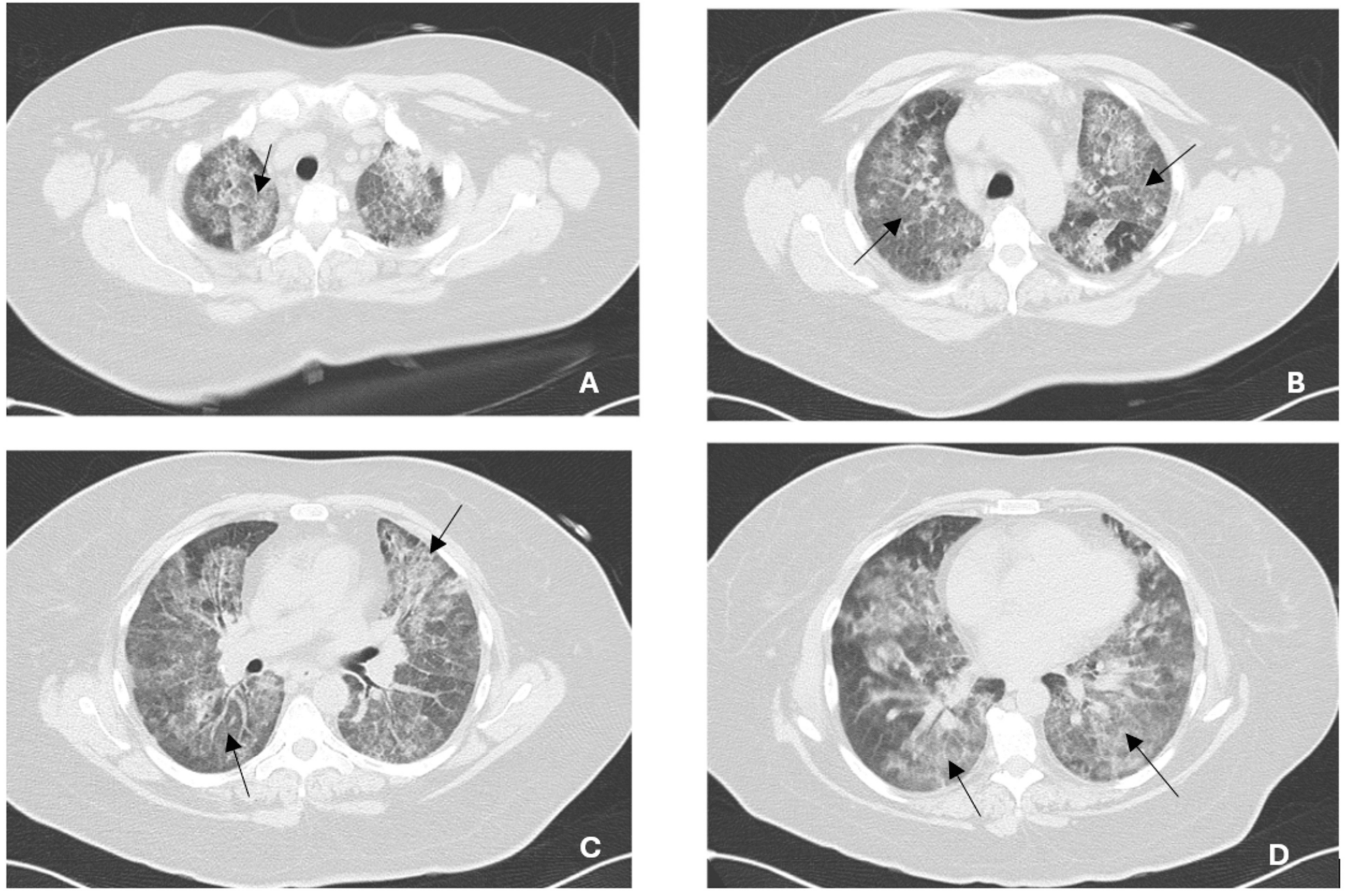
Thoracic CT scan (A, B) Pulmonary apices. (C, D) Lower pulmonary lobes. All images show extensive areas of ground-glass opacities.
